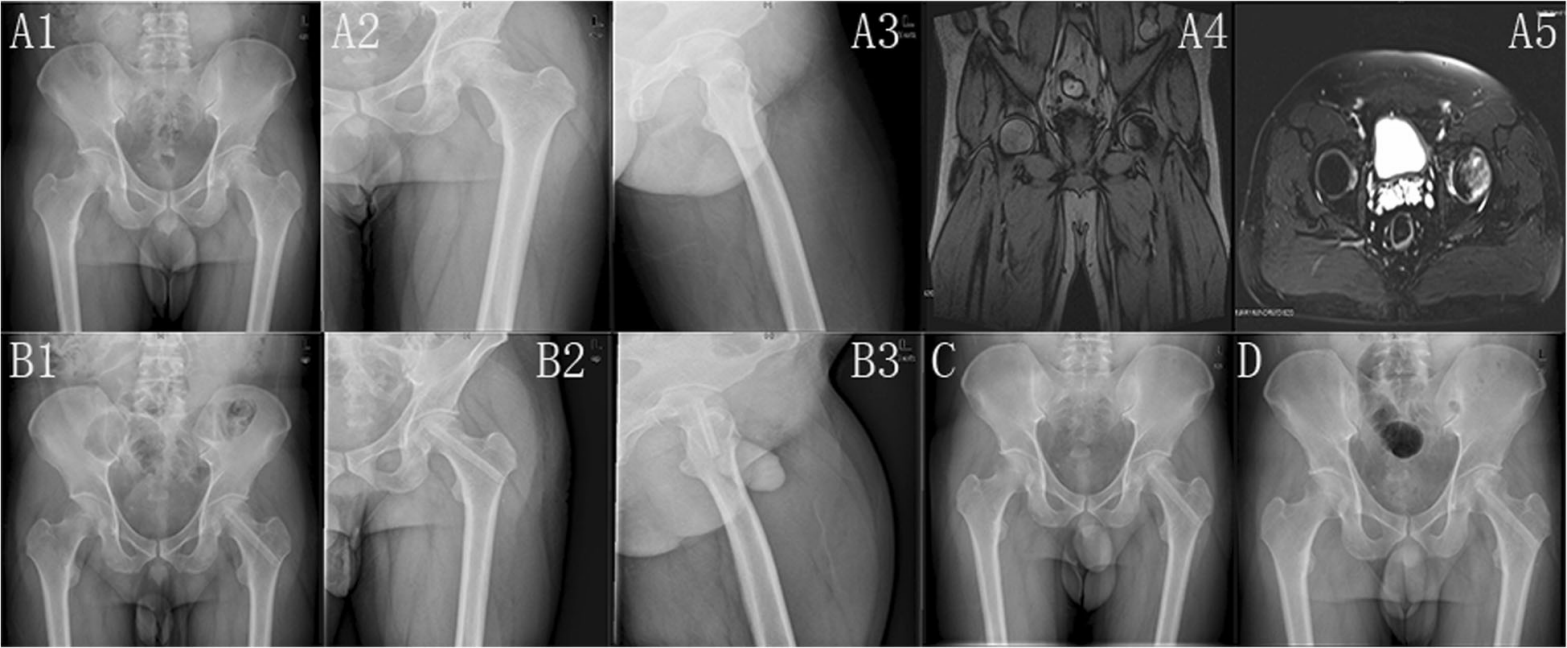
The radiographic outcomes of a 36-year-old man with a long history of drinking and was diagnosed as left femoral head necrosis at Ficat II stage. A1–A5 The patient has early femoral head necrosis evaluated by X film and MRI respectively. B1–B3 The X film of 3 days after the operation. It shows that the femoral head is in normal shape and the grafted fibula integrates tightly in the channel. C, D The images at 1 and 4 months after the operation, respectively. E1–E3 The images at 2 years after the operation. F1–F3 The images at 5 years after the operation, which shows the stability of the articular cartilage and femoral head. G1–G3 The images at 8.2 years after the operation. The femoral head is not progressed and keeps in good shape. The left femoral head was in Ficat I stage and the Harris score was 90 at the last follow-up
